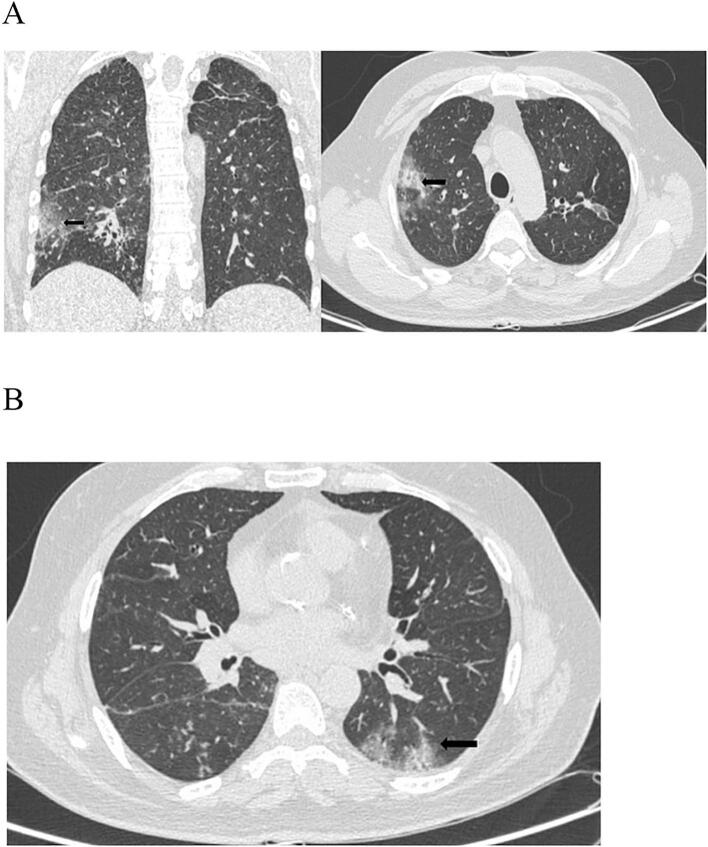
A: Axial non-enhanced CT images (lung window) showing peripheral ground glass opacities in the right upper lobe (arrow). B: Axial non-enhanced CT images (lung window) showing peripheral ground glass opacities in the left lower lobe (arrow).
